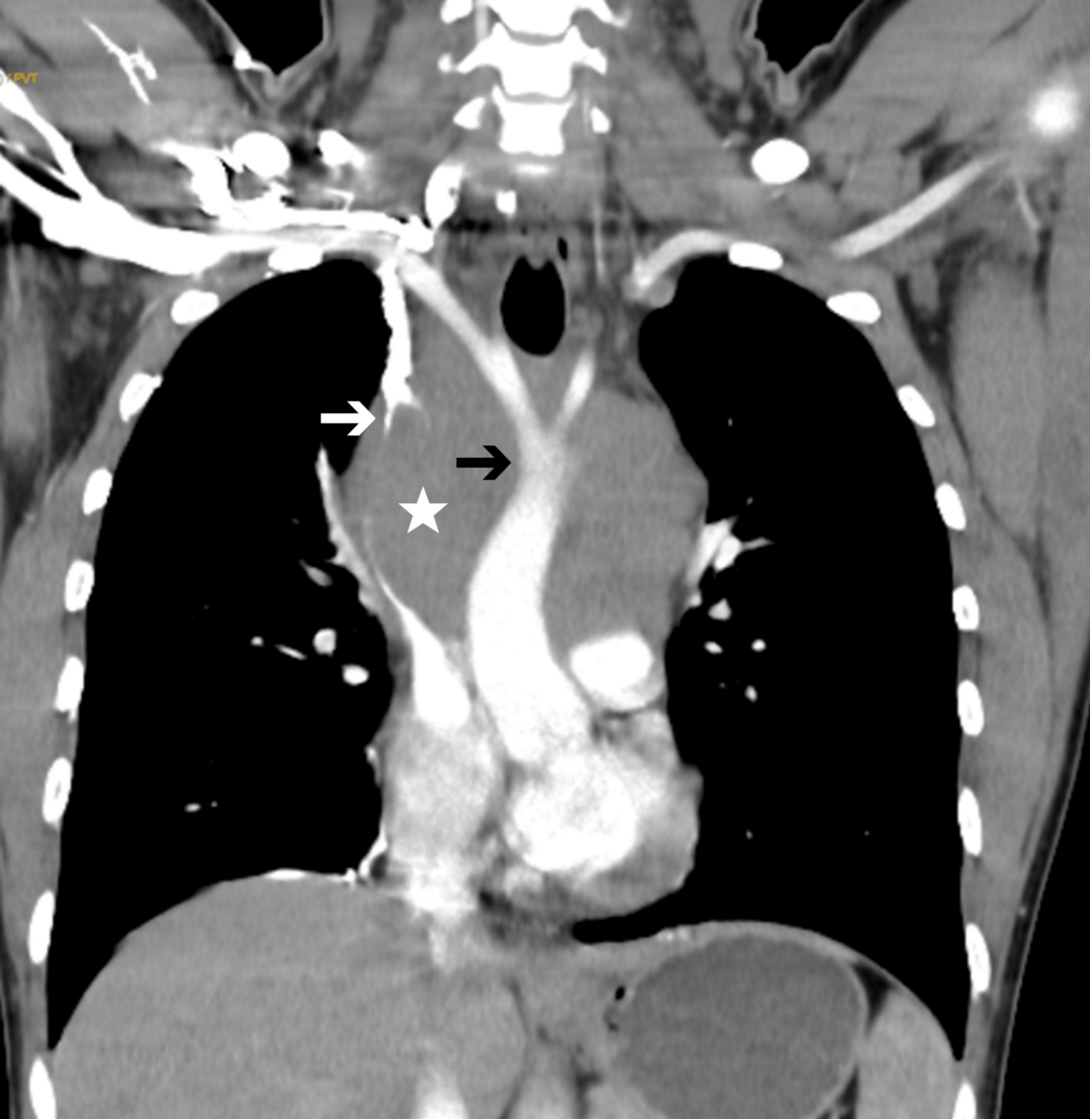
Thorax CT in coronal view showing a large superior mediastinal mass (white star), completely encasing the aorta (black arrow) and causing superior vena cava obstruction (white arrow).
